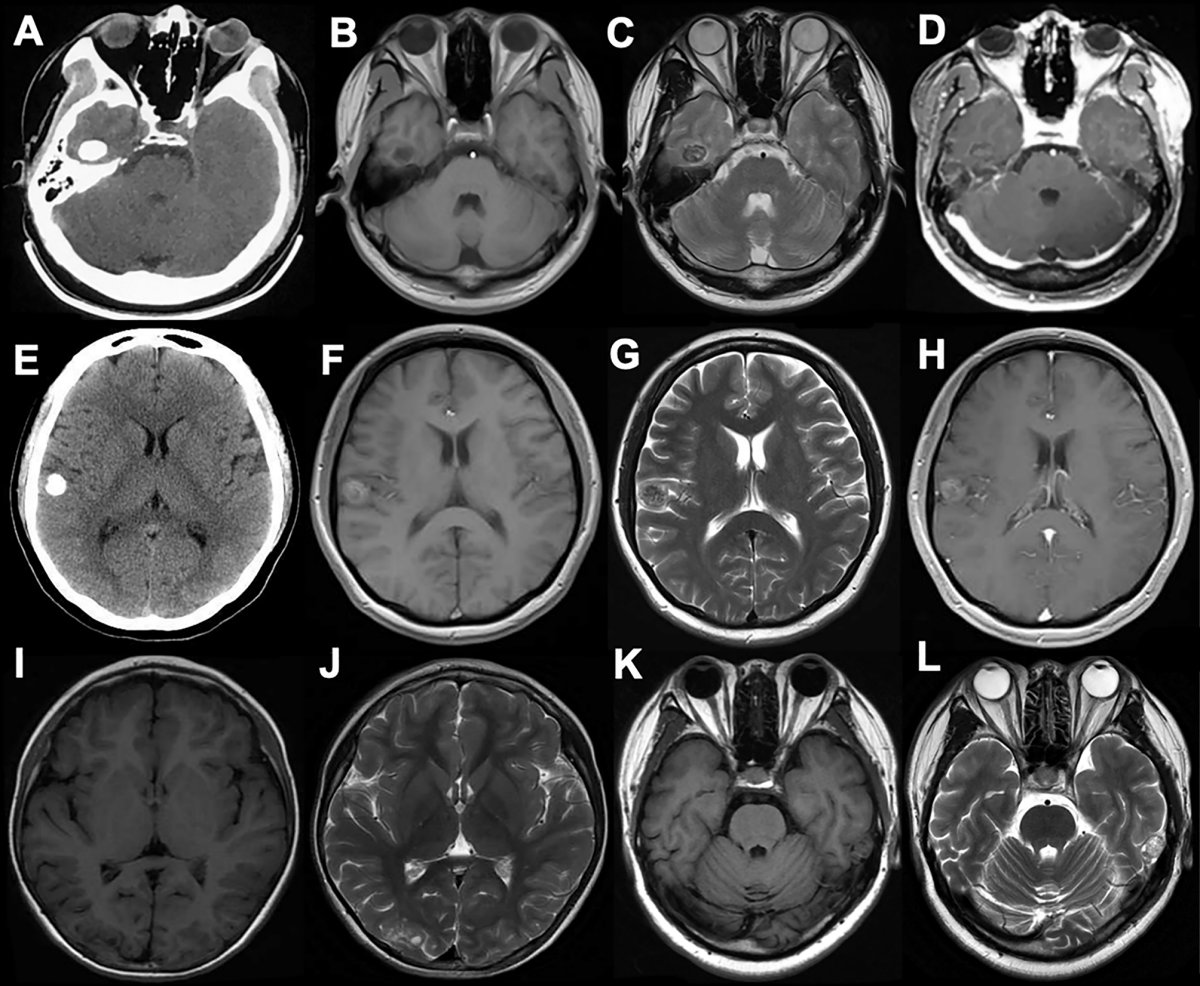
The CT, MRI manifestation of PLNTY. Dense calcifications were often demonstrated in CT images (A, E). The MRI scans often appeared as iso- or low signal in T1W1 (B, I, K), and also can be slight hyperintense because of the composition of calcium salts (F). The increased or mixed-signal were seen in T2W2 (C, G, J, L), and the cystic components were also seen in (J, L). No enhancements were shown in post-gadolinium T1-weighted MR images (D, H).
